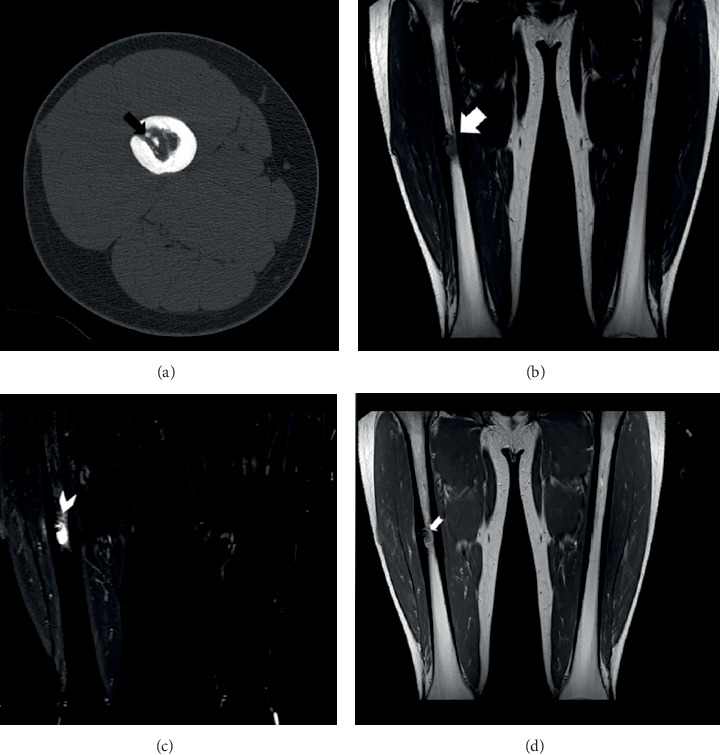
Axial CT image (a) at three months post-RFA, revealing the existence of dystrophic calcifications (black arrow). Coronal MRI scan images of the diaphysis of the right femur of the patient at three months post-RFA, depicting a well-defined lytic lesion with central necrosis. The necrosis had an intermediate to low MR sign on T1WI ((b), white arrow) and prominent surrounding oedema on STIR ((c), arrowhead). After the intravenous administration of contrast media, the lesion showed enhancement only at its periphery ((d) short arrow).
